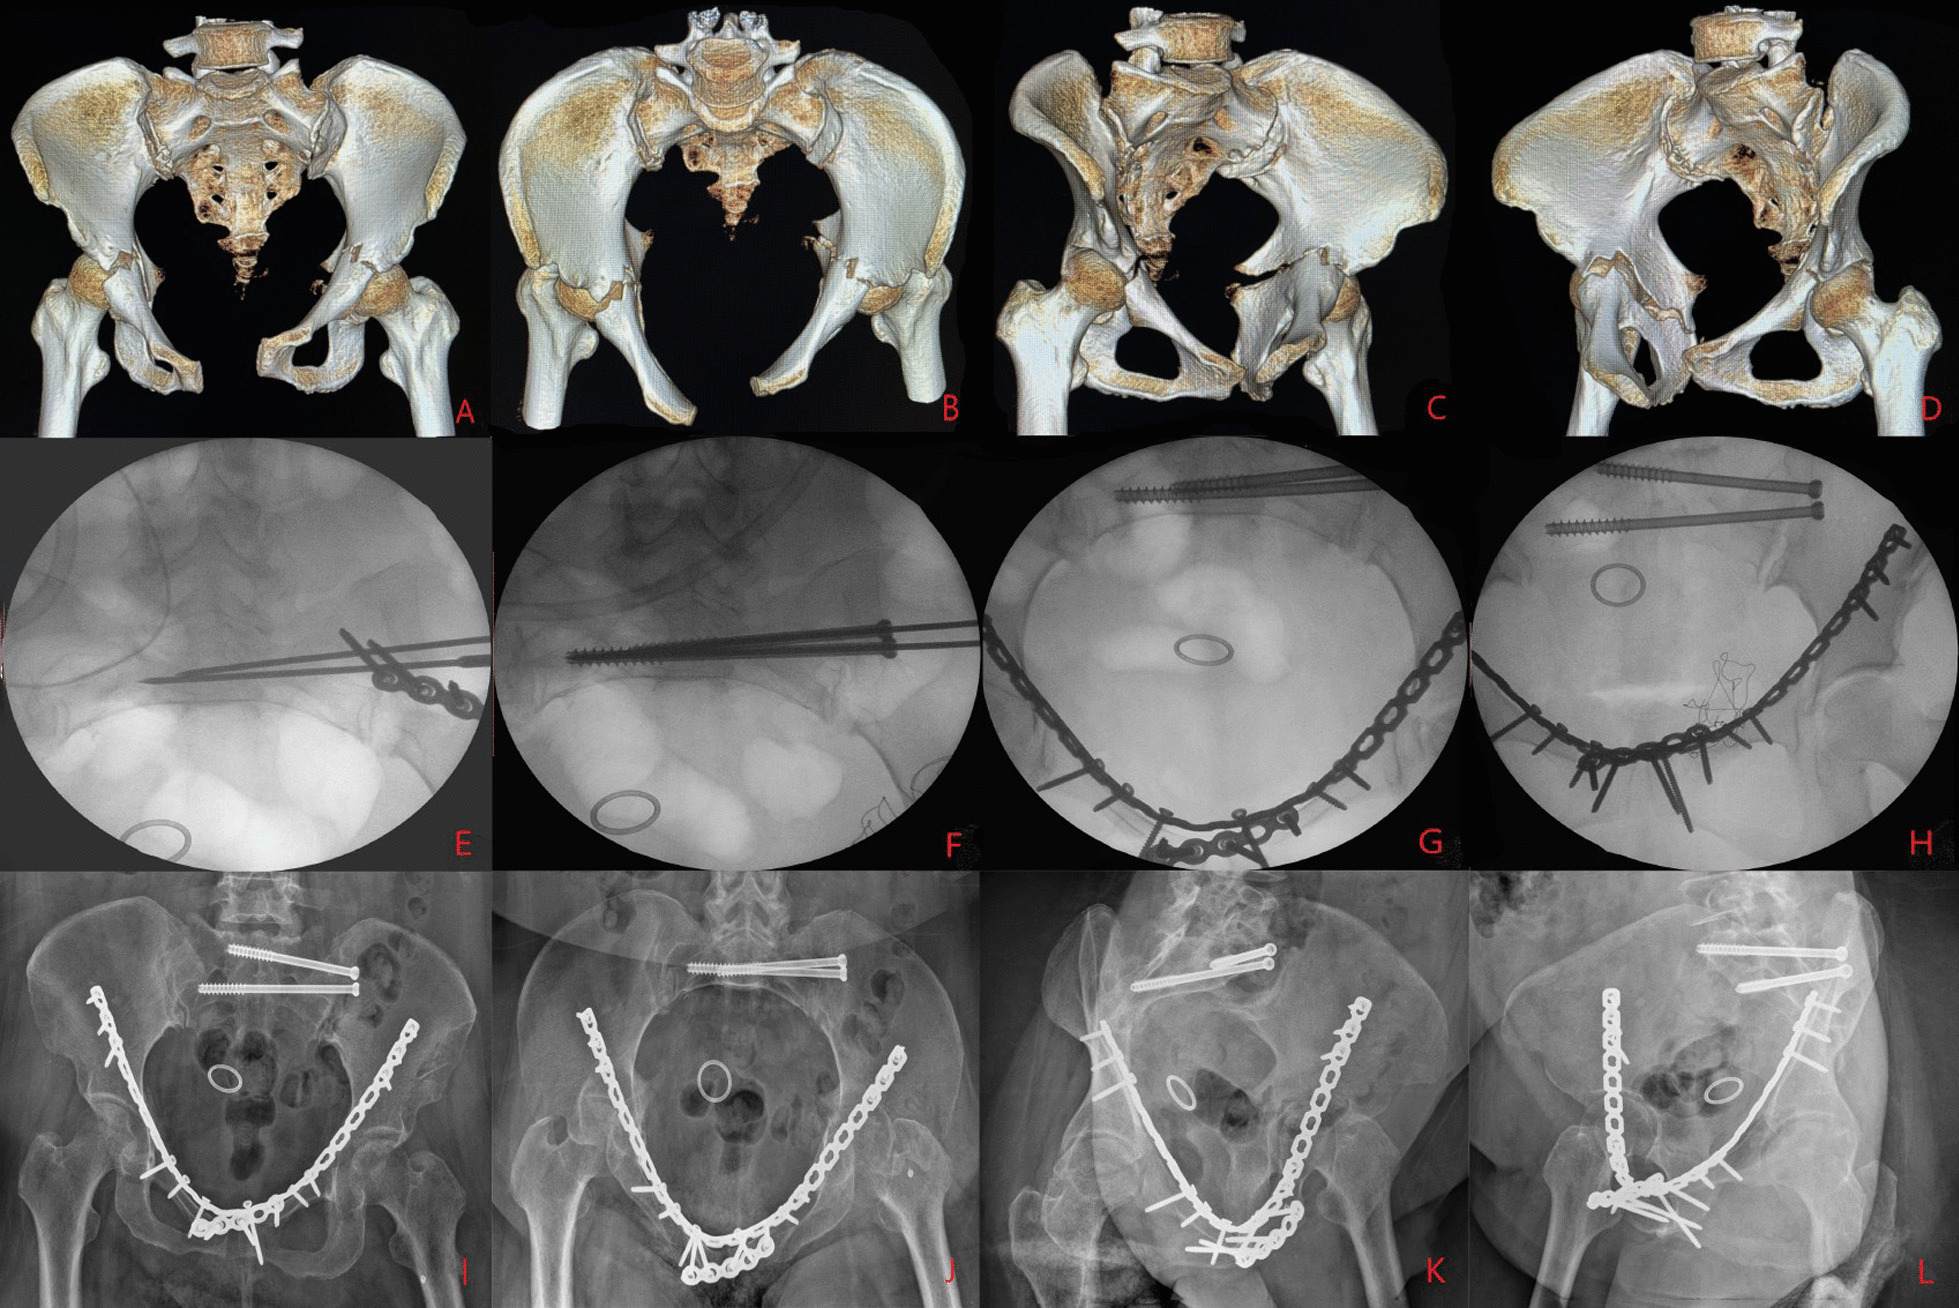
A 56-year-old female patient had an APC III pelvic fracture combined with bilateral transverse acetabular fractures due to a motor vehicle accident (A, B, C, D) and underwent surgery on the 6th day after the injury. A modified Stoppa approach with a small incision in the left iliac fossa was used to first reposition the sacroiliac joint and temporarily fix it with a small plate (E), followed by the placement of two sacroiliac screws (F) and finally repositioning of the bilateral acetabulum with an additional small incision in the right iliac fossa (G, H). The postoperative X-ray film showed excellent reduction in the pelvic fracture and good reduction in the acetabular fracture. The X-ray at half a year (I, J, K, L) after surgery showed fracture healing
